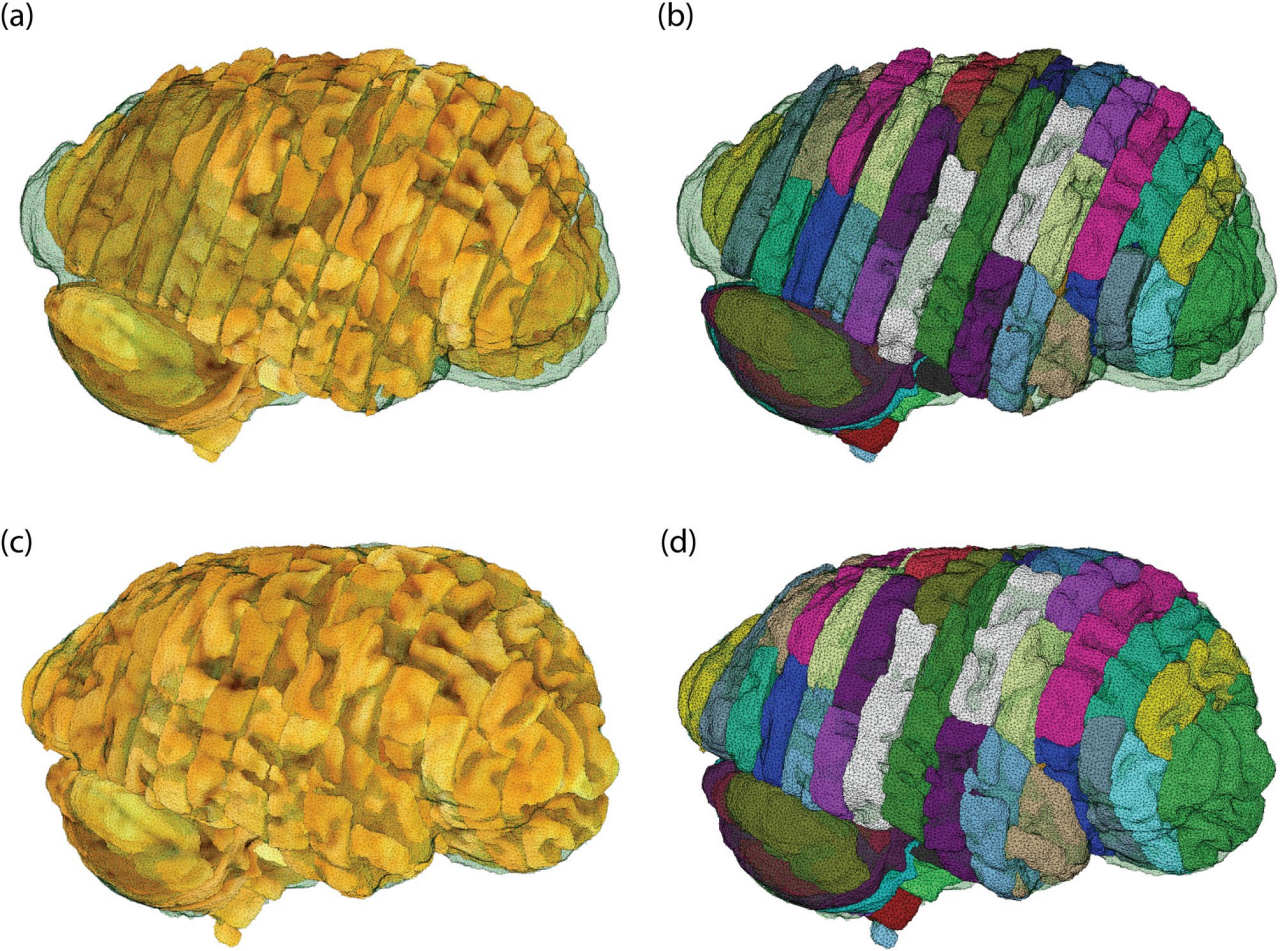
Initialization and refined blockface mosaics. A surface rendering of the whole brain mask derived from the MRI is overlaid in green. (a) Blockface volume for the whole hemisphere resulting from the initialization with the slice photographs; the color for rendering is taken from the blockface photographs. (b) Same volume as (a), where each block is rendered in a different color. The color coding is random and simply emphasizes the block cutting profiles. (c,d) Same volumes as (a,b) after joint registration.
